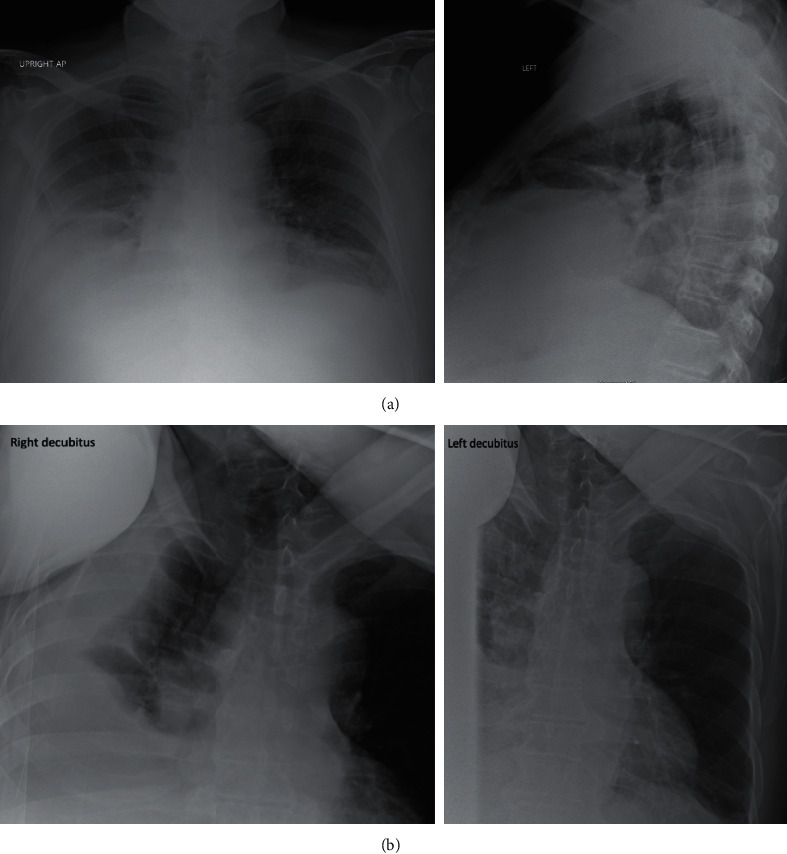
(a) Portable upright chest radiography showing blunting of the right costophrenic angle consistent with small-to-moderate right pleural effusion and linear meniscus with compression atelectasis. (b) Chest X-ray in right decubitus position, showing a large, layering pleural effusion on the right with a possible location at the right lung base.
